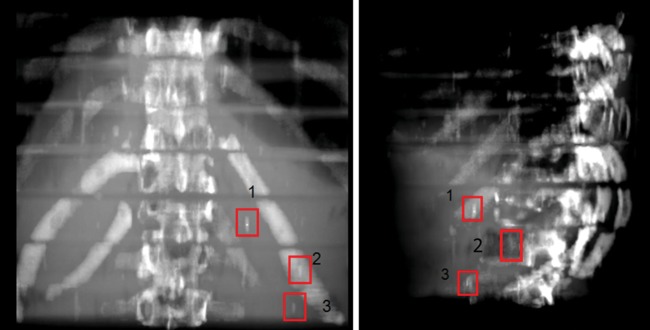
Digitally reconstructed radiographs (DRRs) of the previous CT scan (see Figs 4 and 5). Marker positions: 1 = Visicoil: 0.35 x 20 mm, 2 = Beammarks: 1.2 x 5 mm, 3 = Carbon marker, 1 x 3 mm.
